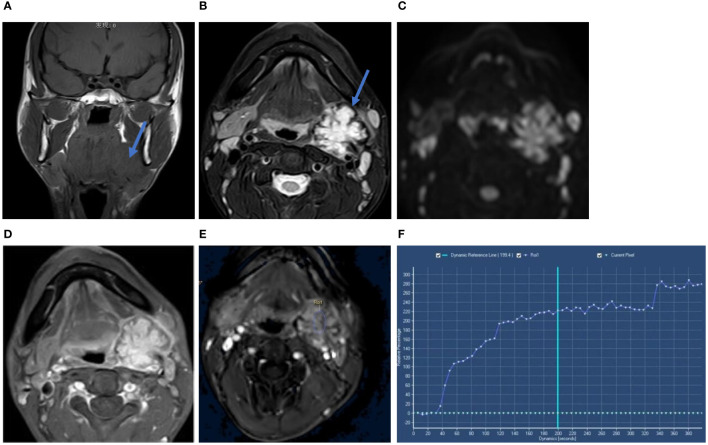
Coronal T1WI scan (A) showed the tumor mass on the upper pole of the left submandibular gland (blue arow). Axial T2WI-FS (B) showed heterogeneous hyperintense with a hypointense lobulated rim (blue arow). The tumor showed heterogeneous high signal intensity on DWI (C). Axial gadolinium-enhanced T1-weighted image (D) showed heterogeneous enhancement with a hypointense rim. Time-intensity curve (TIC) was progressive ascending type (E, F). Its x-axis represents the time of the enhanced scan, from 0 seconds to 380 seconds, with the extension of time, the curve is on the rise.
